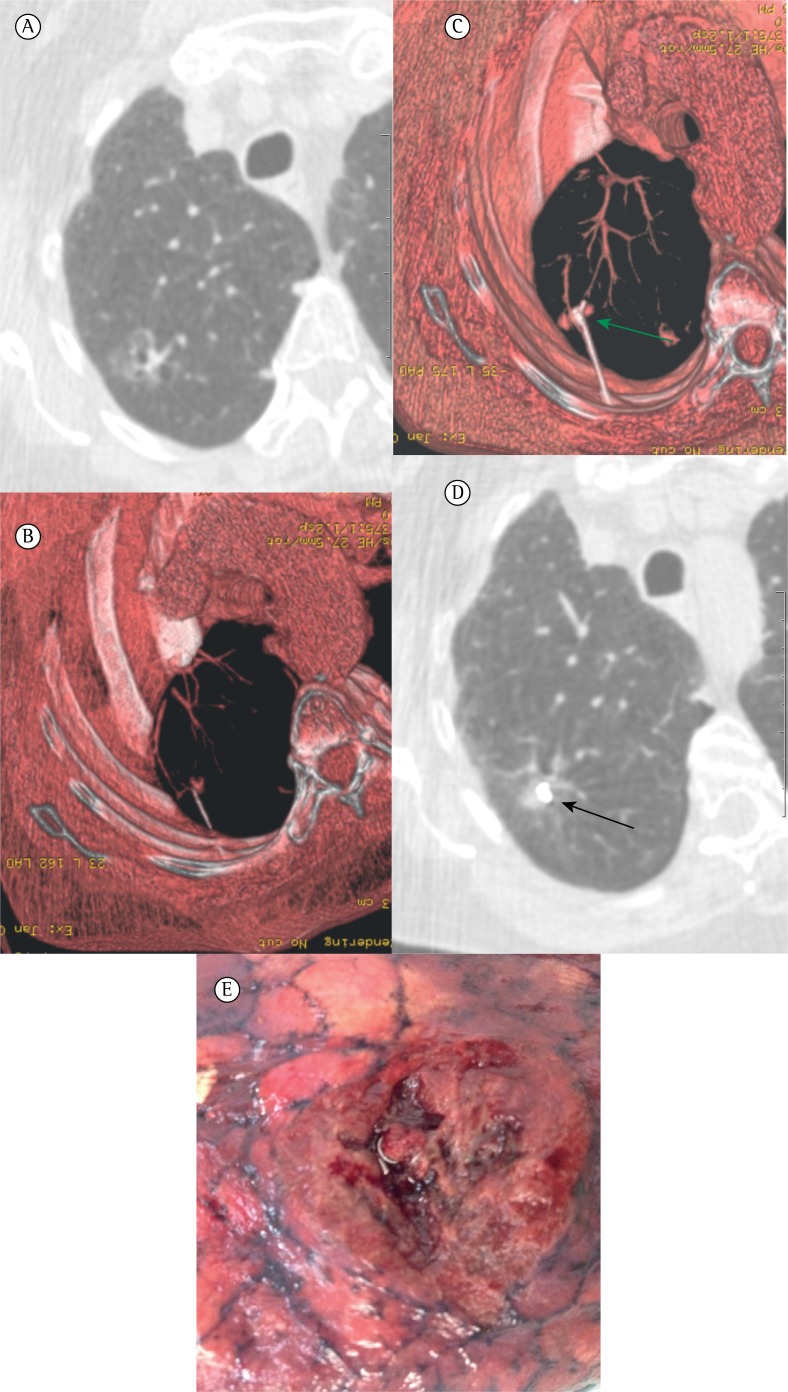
In A, a CT scan demonstrating a 1-cm ground-glass nodule in the central
